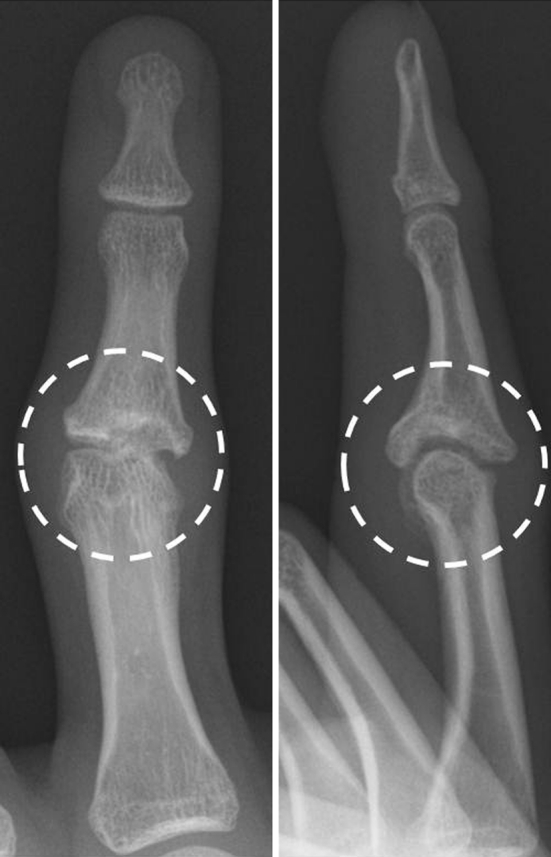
X-ray image before treatment. *Posterior* and *anterior view* show bone defect in the articular surface of the PIP joint of the middle phalanx and displacement of the middle finger to the ulnar side. *Lateral view* shows similar findings
